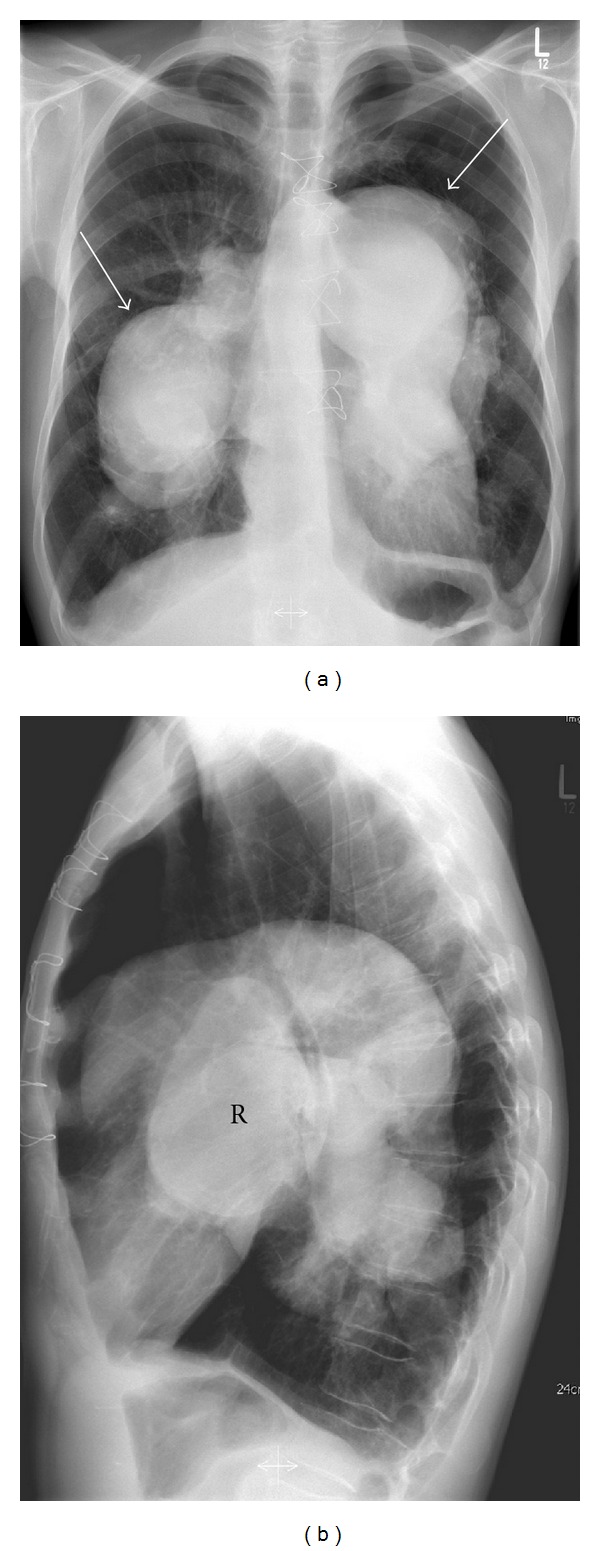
Chest radiographs ((a) and (b)) from 2012 showed severe dilatation of both pulmonary arteries (arrows). (R = right pulmonary artery).
